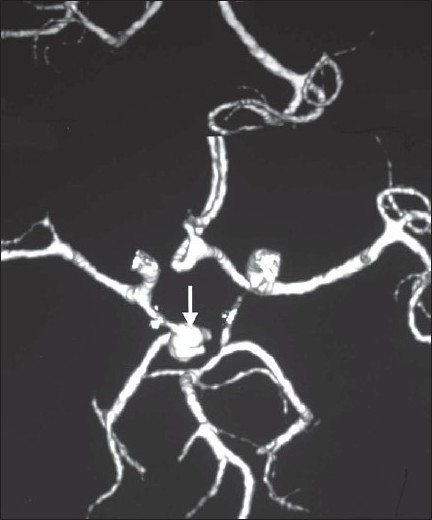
Three-dimensional reconstructed CT angiogram shows fusiform aneurysm with a saccular projection arising from the middle of right fetal posterior cerebral artery (PCA) and directed posterosupero- laterally (white arrow)
